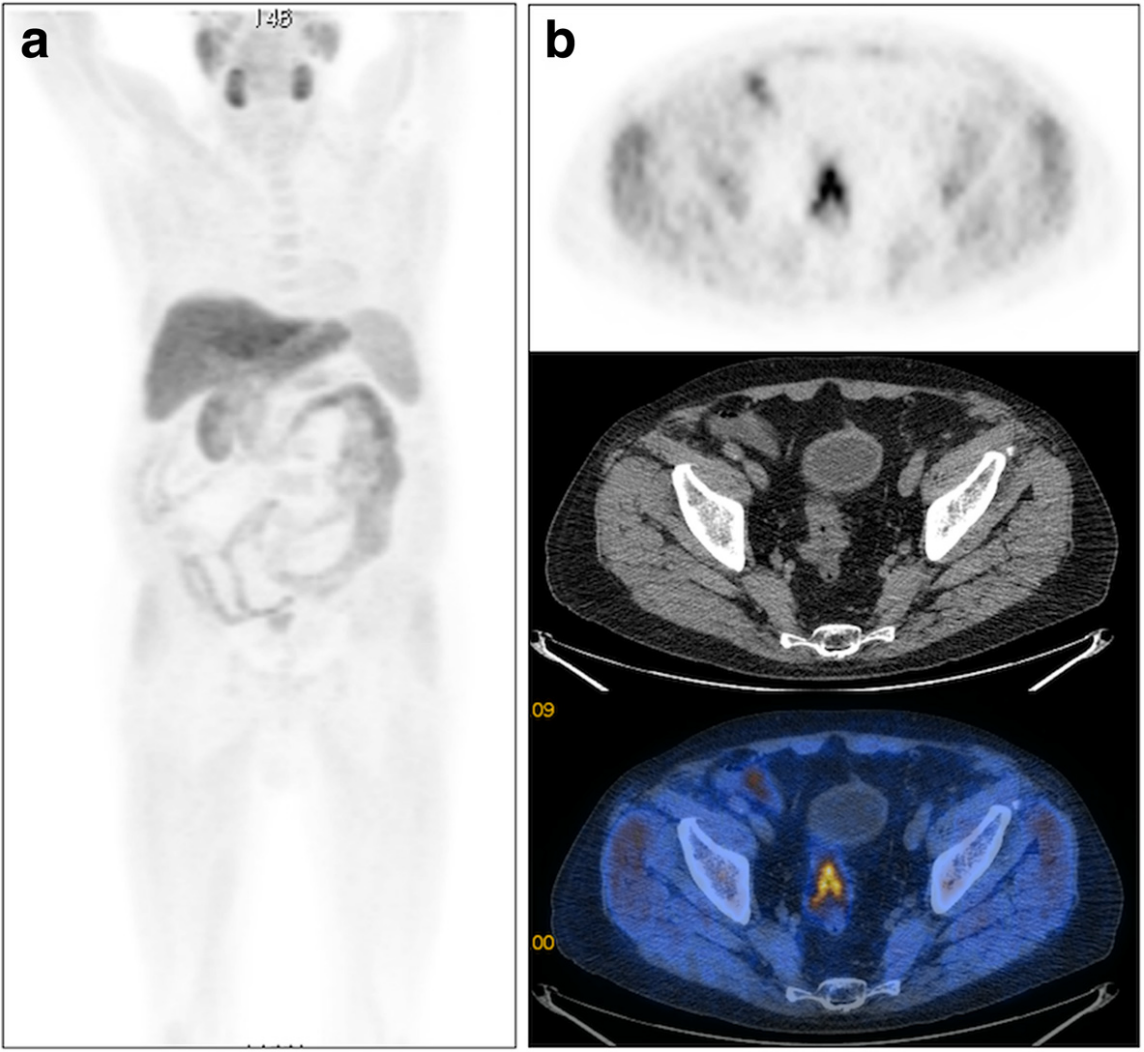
Case#7, [^18^F]fluorocholine PET/CT in a 80-yr old man treated with increased PSA after radical prostatectomy for prostate cancer. MIP (a) and axial (b) image show a metabolically active area in the rectum which was subsequently diagnosed as colorectal adenocarcinoma (pT3N0)
